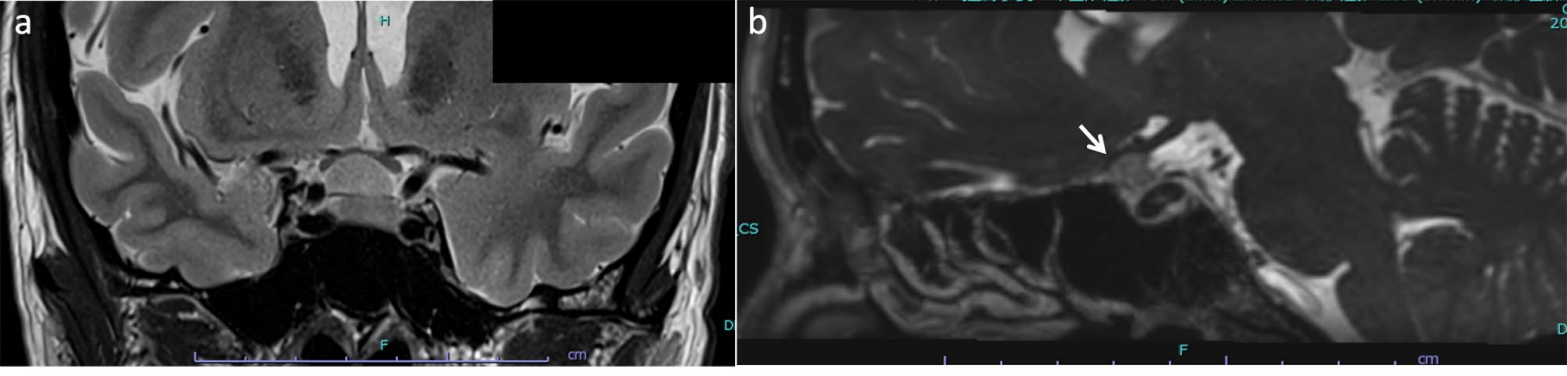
MRI of the brain. A: Coronal T2-weighted image demonstrated the suprasellar mass compressing the optic chiasm upwards, especially on the right side. B: Post-contrast sagittal image on CISS demonstrated the enhanced mass compressing the anterior chiasm (arrow)
